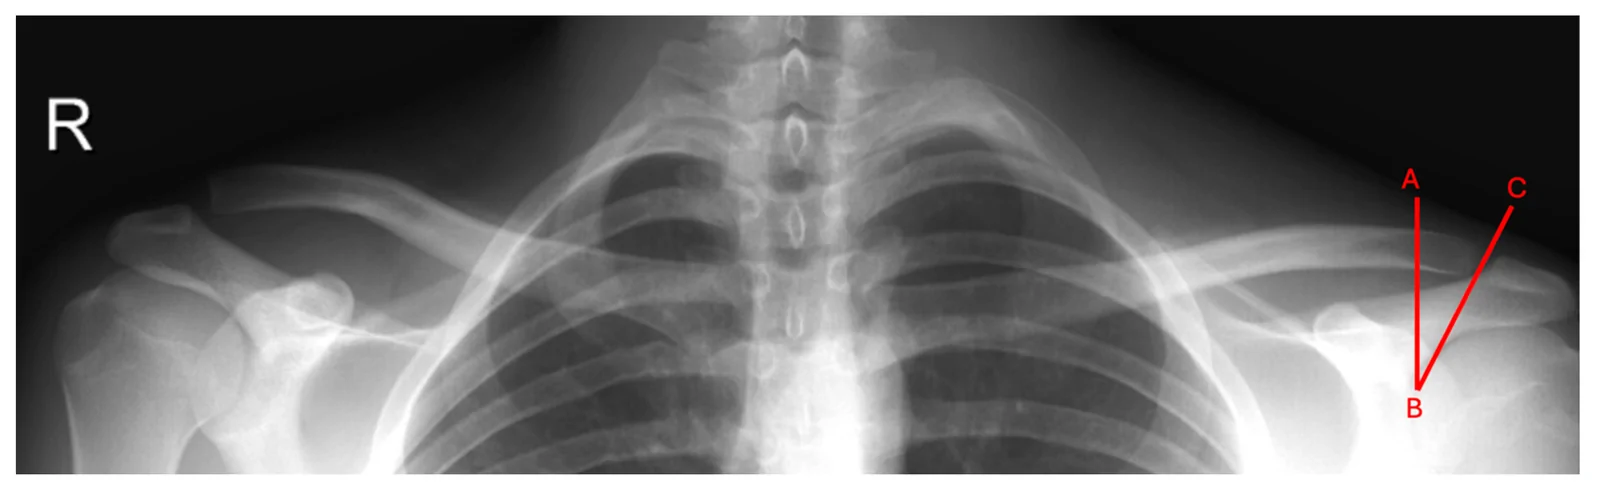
Measurement of the acromioclavicular joint coronal orientation angle (AJCO). The superior and inferior endpoints of the AC joint space were identified and connected to define the AC joint orientation line (B–C). The radiographic vertical reference line (A–B) was constructed on the standardized bilateral Zanca radiograph. AJCO was defined as the acute angle between these two lines. The injured shoulder is displayed separately on the right to distinguish native anatomy from trauma-related displacement. R: Right.
